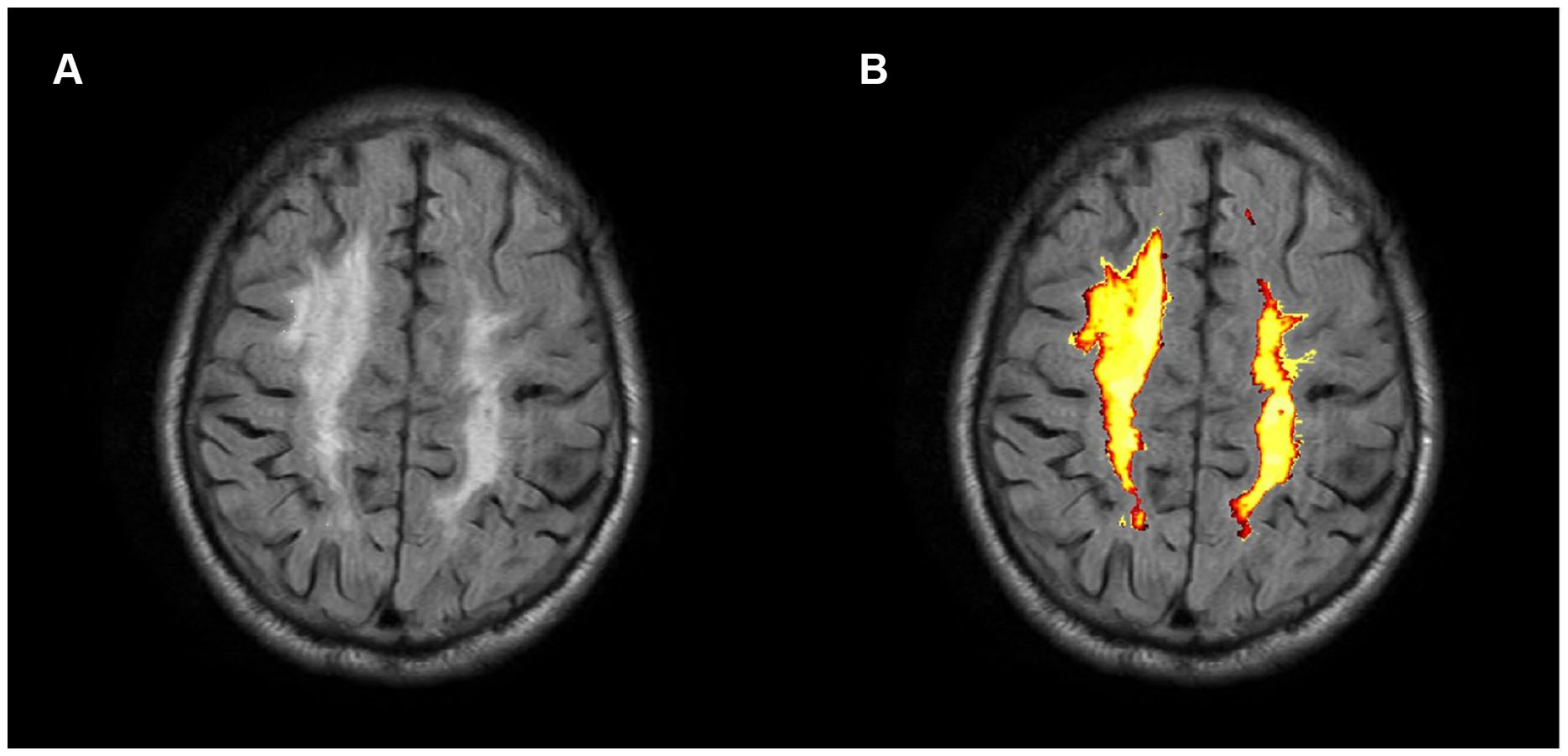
Axial FLAIR image of a CSVD participant (A) and associated probabilistic lesion volume map (B) generated by the LST-LPA.
